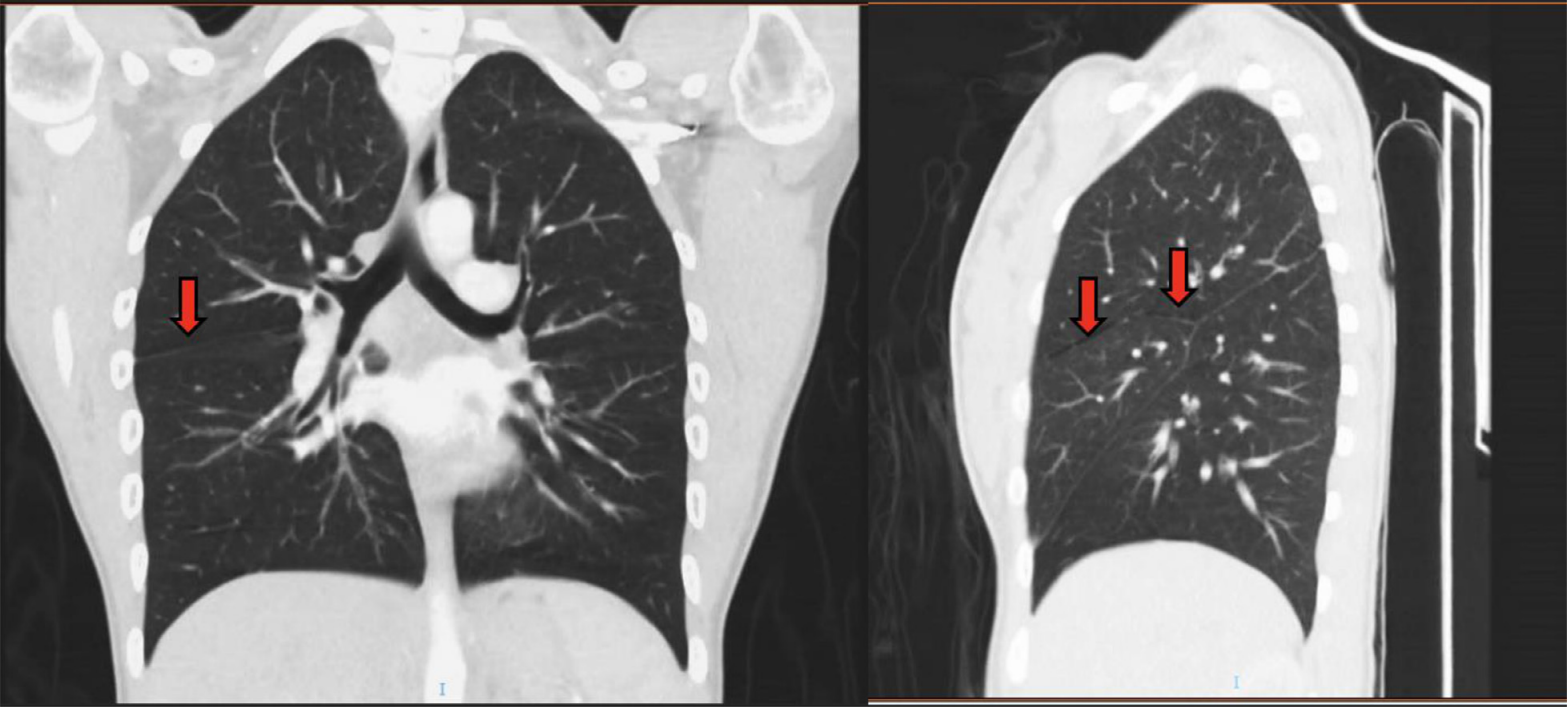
CT angiogram of chest AP and lateral views - curvilinear streak seen immediately adjacent to the course of the minor fissure of the right lung as marked by the red arrow.
